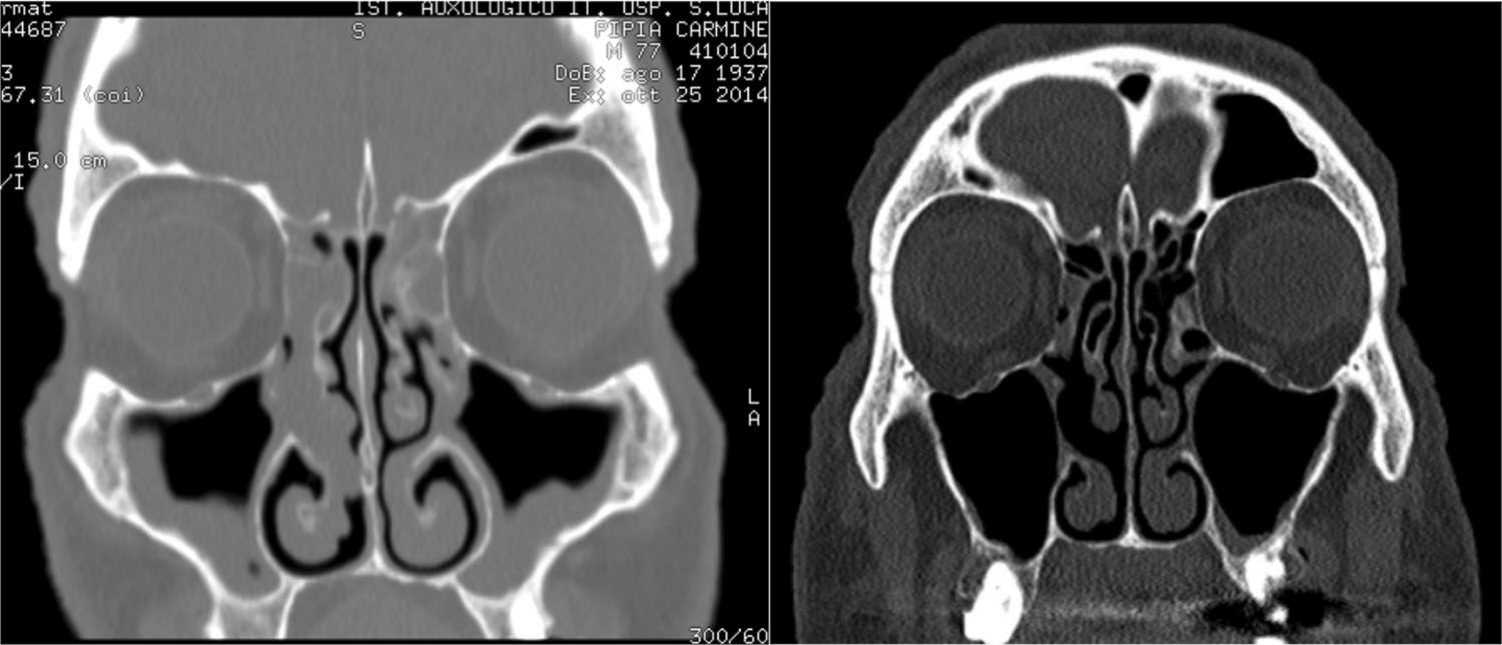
Example of a CT scan’s patient at T0 (on the left side) and at T4 (on the right side) of dupilumab treatment
